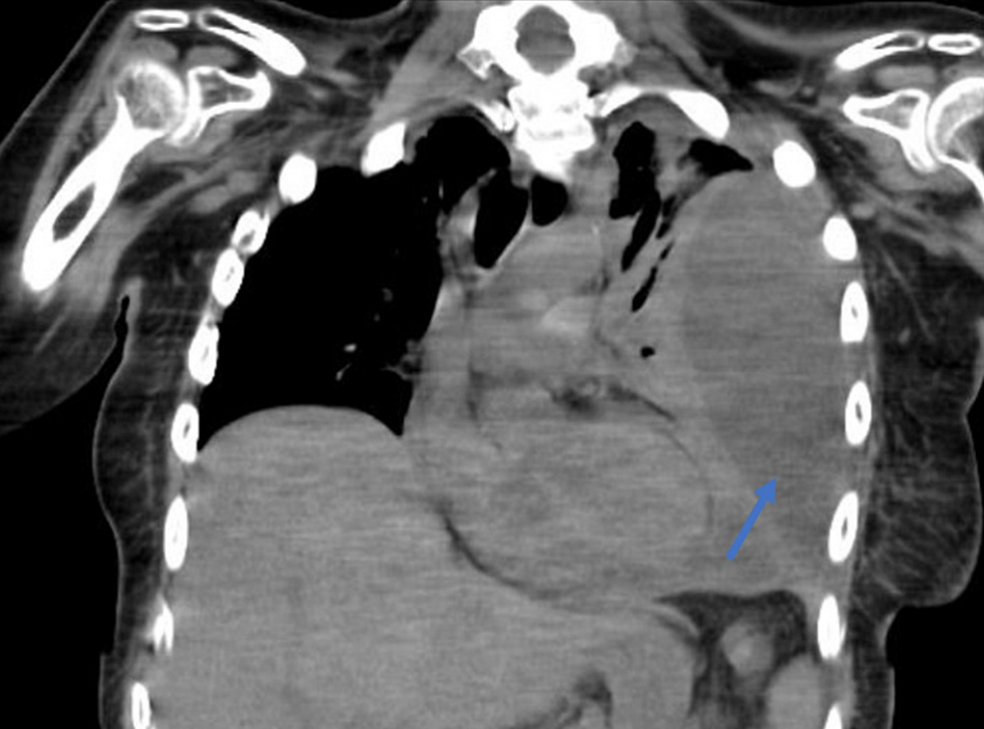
CT scan (coronal view) showing a left pulmonary empyema (blue arrow).
